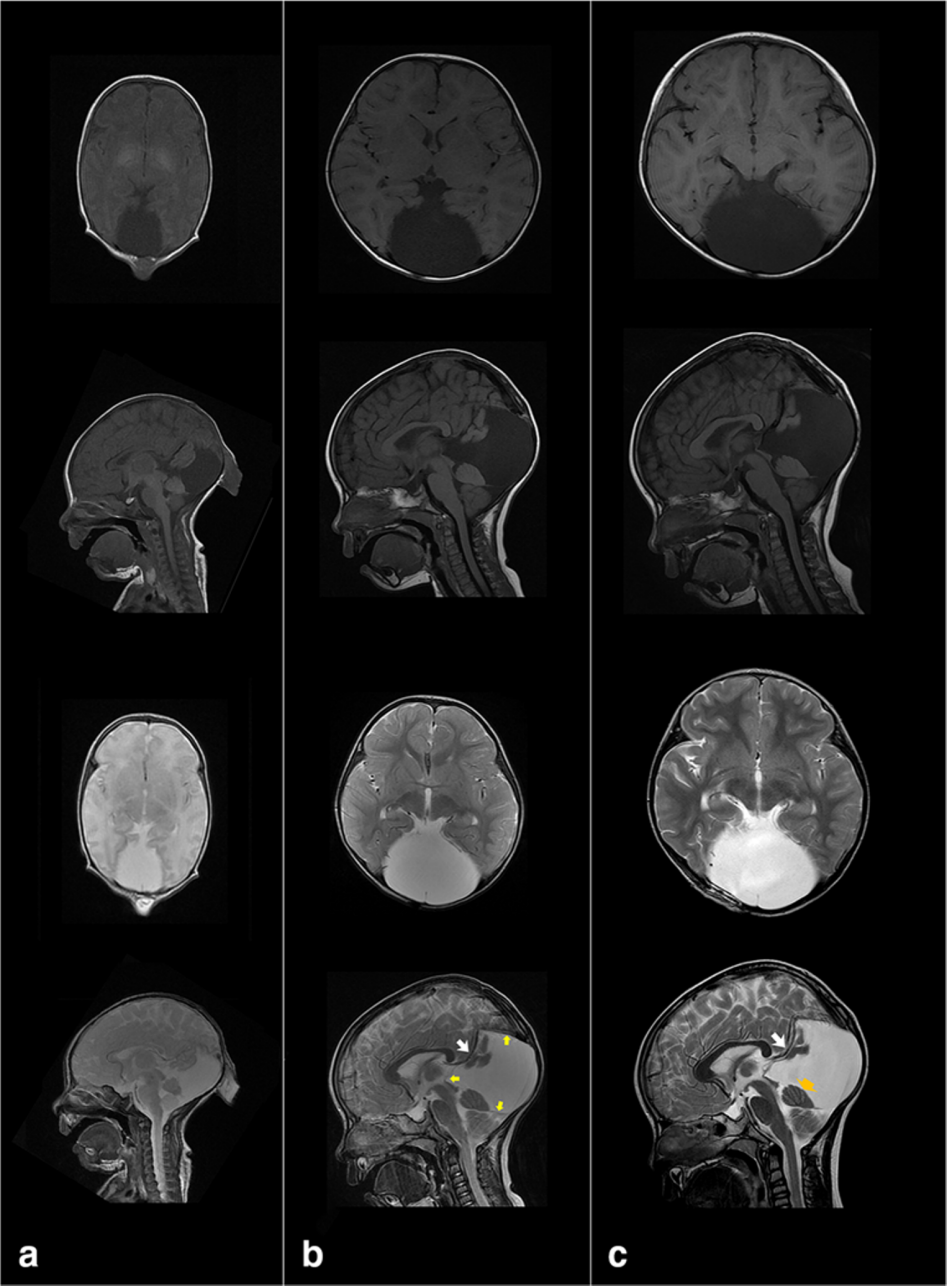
Preoperative MRI scan. a Axial and sagittal T1-weighted or T2-weighted image at birth showed the lack of cranium and encephalocele. Intracranial structures appear to protrude to outside of the cranium with the arachnoid membrane. b MRI at age 1 year and 10 months. Although subarachnoid cyst remained and gradually increased (small yellow arrow), there were no neurological symptoms. c MRI at age 4 years and 2 months. Axial and sagittal T1-weighted or T2-weighted image indicated enlargement of subarachnoid cyst not only horizontally but also vertically. The cyst compressed the bilateral cerebellar hemisphere from above (orange arrow). Sagittal T2-weighted image clearly shows an abnormal blood vessel continuous with the internal cerebral vein suggesting falcine sinus (white arrow, b and c)
